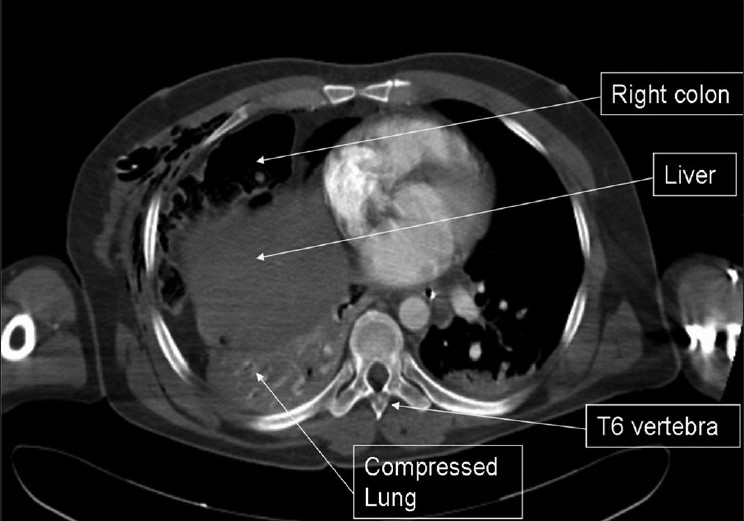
Axial computed tomography scan demonstrating right colon and right liver lobe in the right chest cavity with compression of the lung parenchyma
